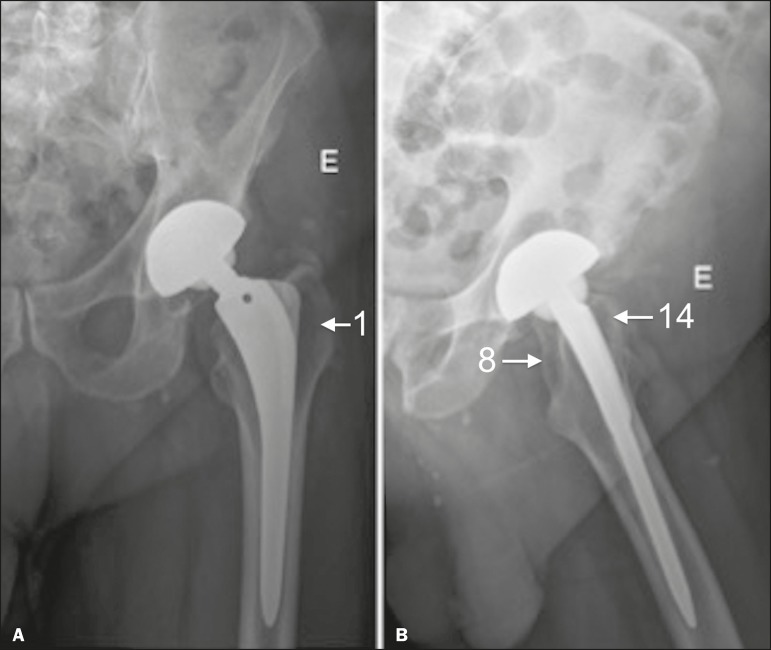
Anteroposterior and lateral X-rays of the left hip (A and B, respectively), showing osteolysis in femoral zones 1, 8, and 14 (arrows).
